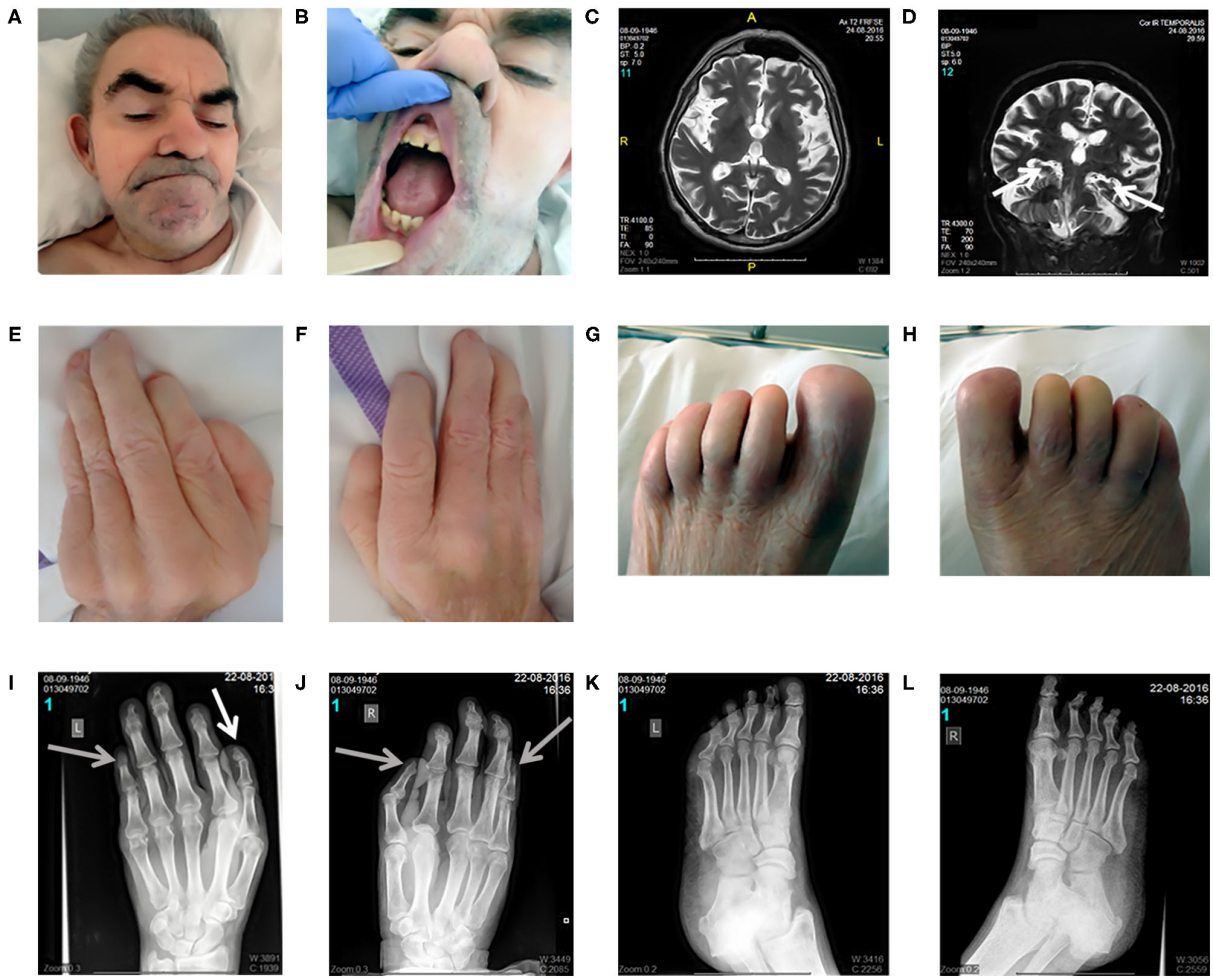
Clinical and radiomorphological characteristics of an aged case of DOORS syndrome. Craniofacial dysmorphism (coarse facies, hypertrichosis, broad nasal bridge, wide mouth, smooth philtrum, slightly hypertrophic gingiva, widely spaced teeth, and large ears) could be observed distinctively (A,B). Brain MRI revealed mild generalized supratentorial and infratentorial atrophy, predominantly affecting the temporal lobes, including moderate hippocampal atrophy [white arrows; (C,D)]. Regarding limb alterations, onychodystrophy, and abnormal fingers and toes with small distal phalanges were detected (E–H). Accordingly, the X-ray assessment demonstrated the presence of a triphalangeal left thumb [white arrow; (I)] and a triphalangeal-like right thumb, but with suspected synostosis of the distal phalanges. A similar synostosis of the distal phalanges could be observed in the fifth fingers [gray arrows indicate these three synostosis; (I,J)]. Regarding the feet, the middle phalanges could not be distinguished and the distal phalanges of the first toes were smaller than normal (K,L). Written informed consent was obtained from the legal guardian of the participant in the study for the publication of photo materials with identifying information.
